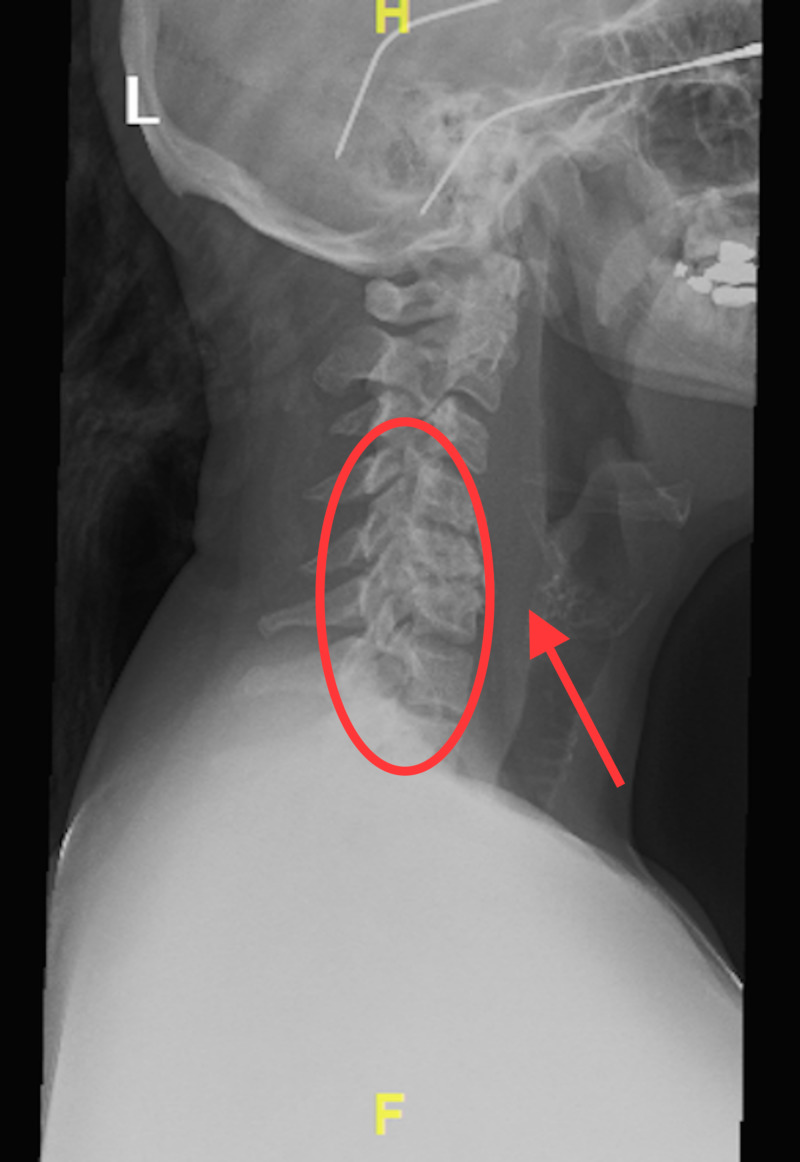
Soft tissue neck X-ray showing degenerative changes (within the oval) of the cervical spine at C4-C5, C5-C6 and prevertebral edema (arrow)
